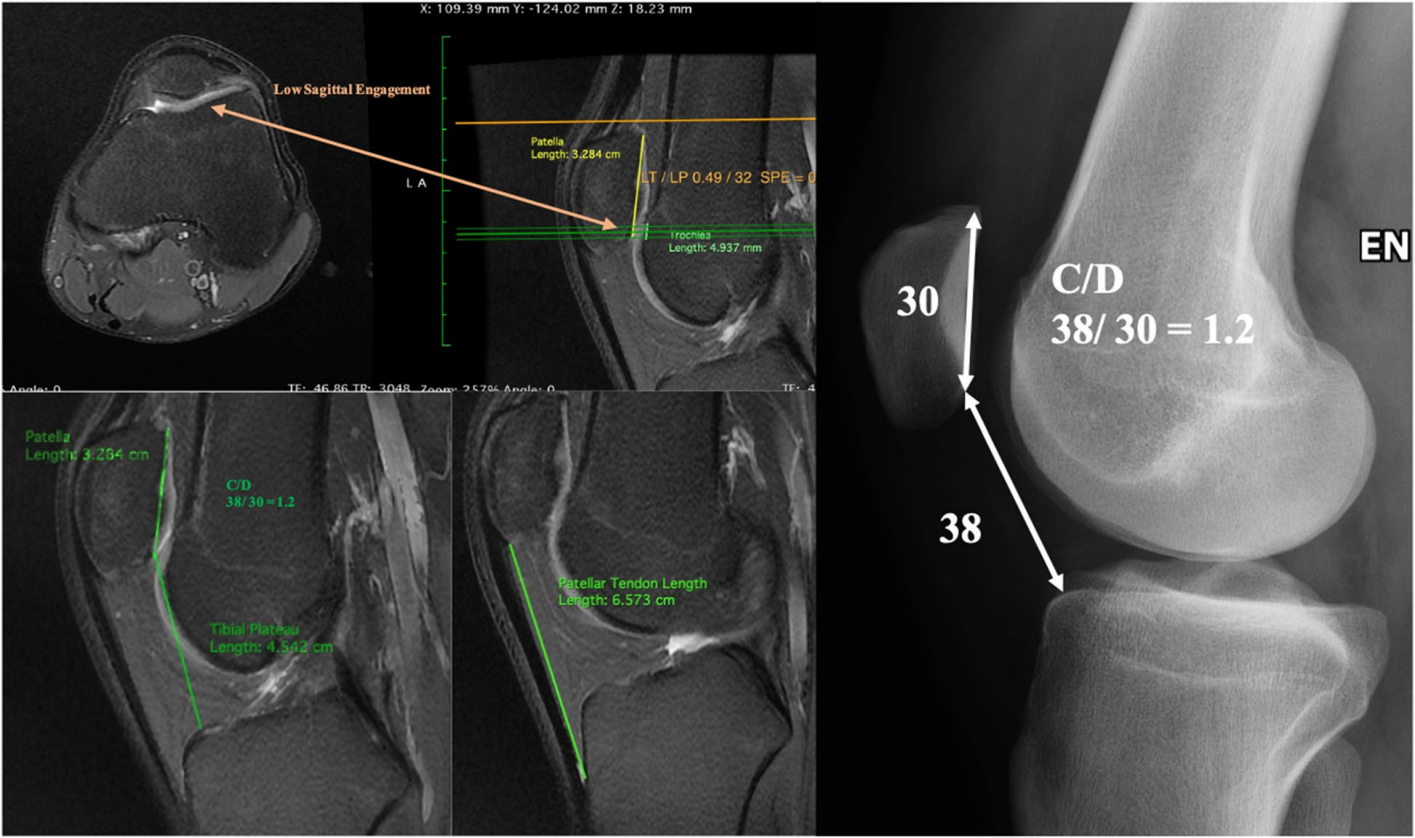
Patella Alta quantification: X-ray with the Caton-Deschamps index, MRI with the Sagittal Patellar Engagement index, notice the patellar tendon length
